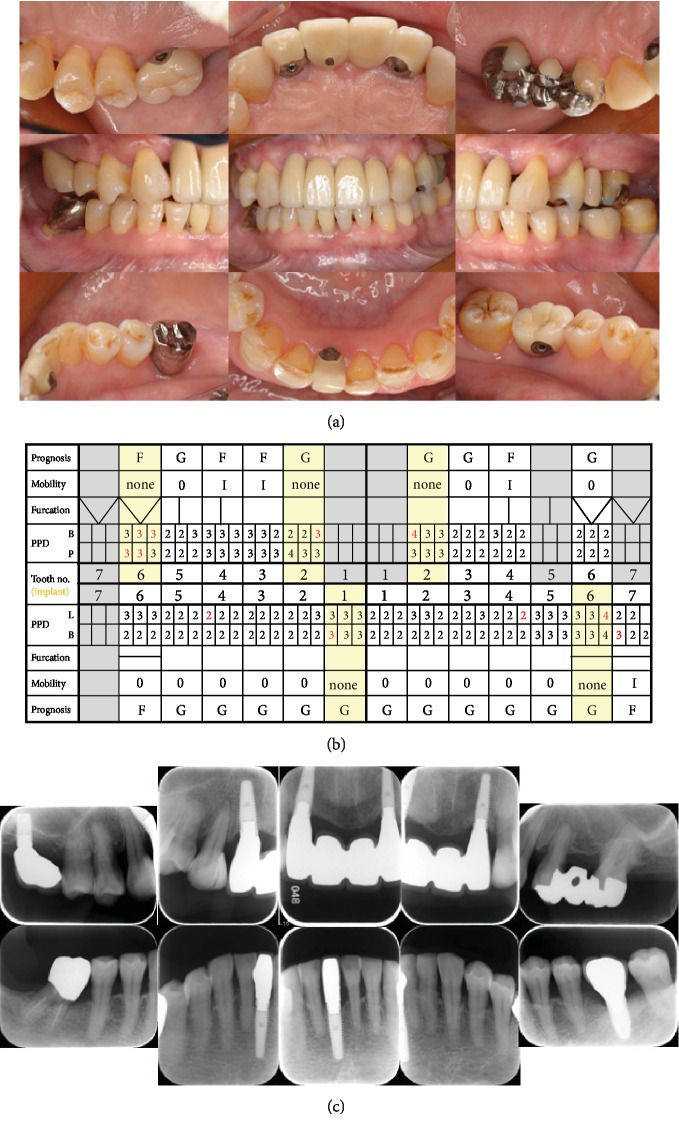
(a) 12-year postoperative intraoral photographs (April 2016). Intraoral view after completion of care. The gingival texture and peri-implant mucosa appear healthy. (b) 12-year postoperative periodontal chart. Prognoses ranged from “fair” to “good” (PPD: periodontal probing depth; yellow: implant). (c) 12-year postoperative radiographs. Posttreatment radiographs suggest improvement of the severe bone resorption lesions. No bone resorption is seen around the five implants.
